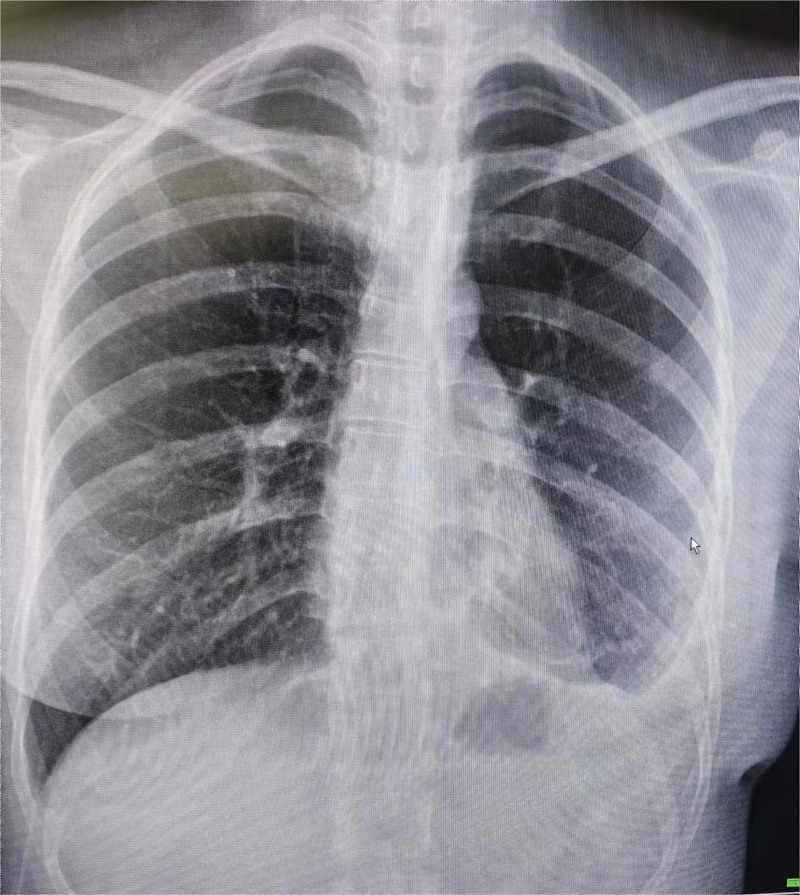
Postoperative chest digital radiography positive performance.
